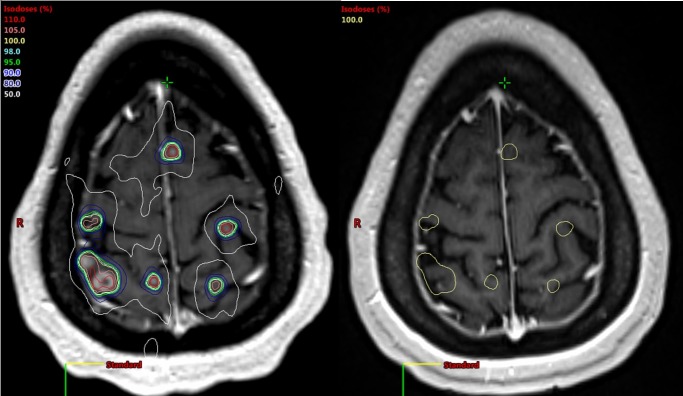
Baseline brain magnetic resonance imaging (MRI) with plan isodose lines, and an 18-month MRI with control of all treated lesions
